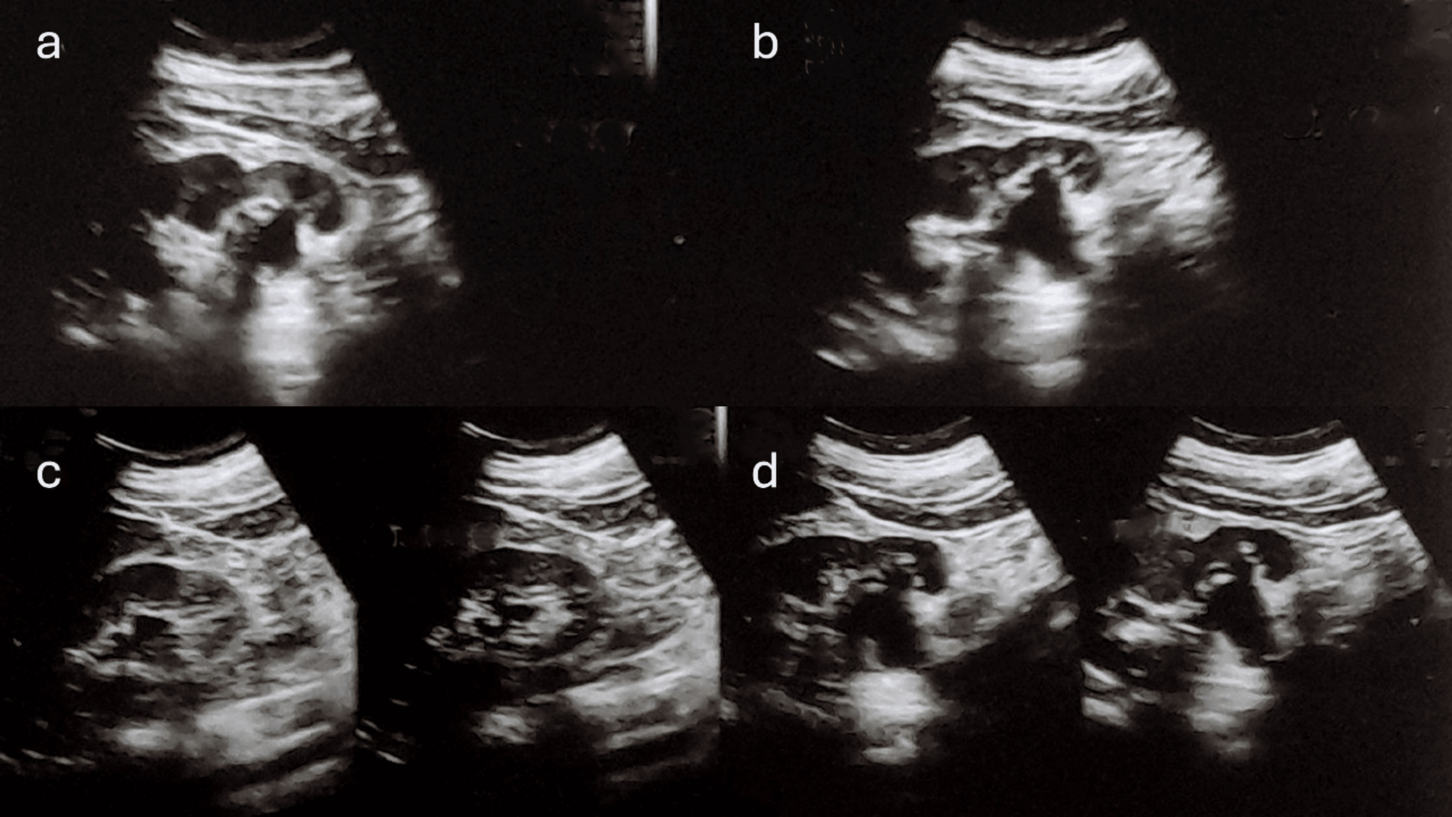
Postoperative ultrasound demonstrating normal-looking bilateral kidneys (a, b, d: left kidney; c: right kidney).
